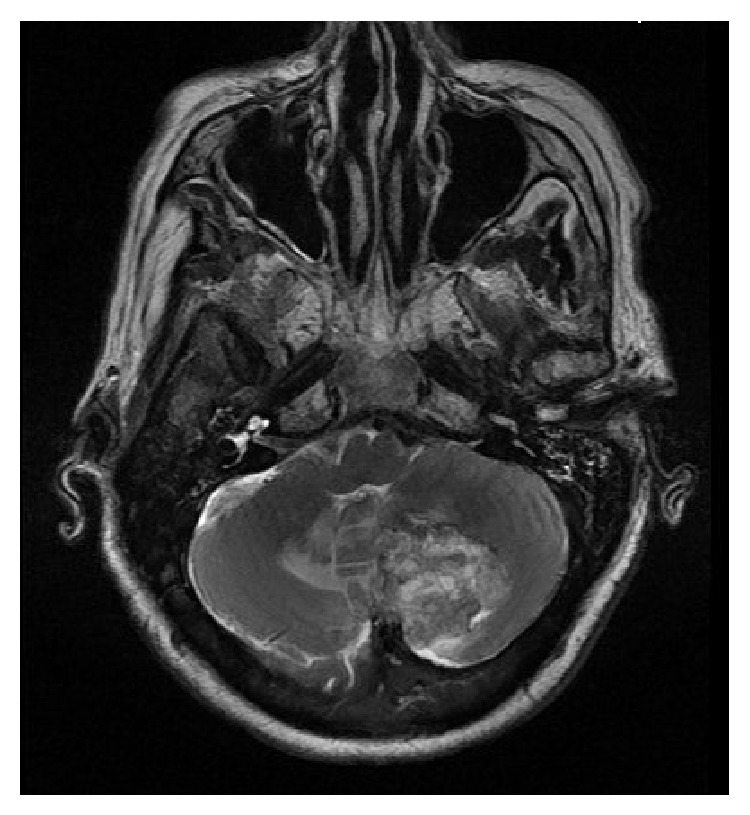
Magnetic resonance imaging of brain (axial view) showing a lobulated heterogeneous mass involving the medial left cerebellar hemisphere, measuring 4.0 × 3.5 × 2.5 cm associated with edema crossing the midline to the right cerebellar hemisphere, and compressing the fourth ventricle (the first case).
